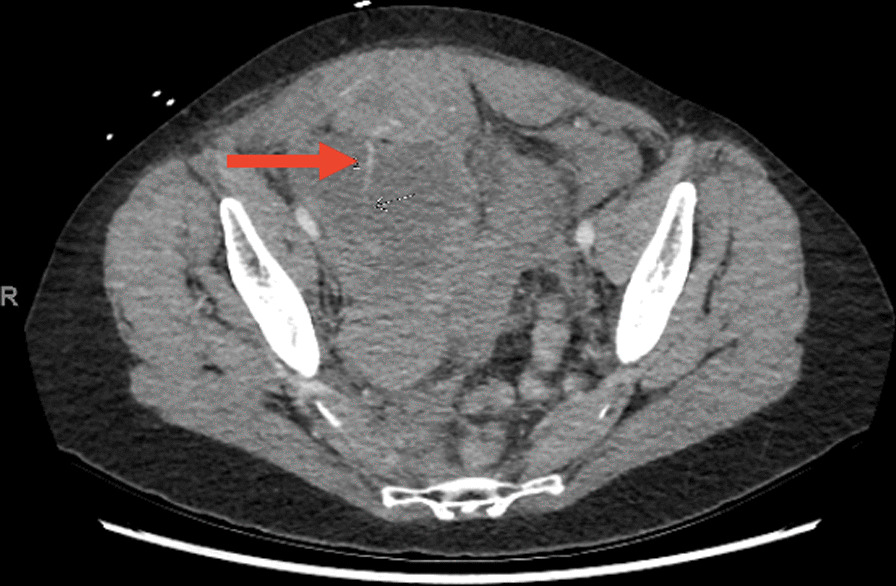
Axial slice of arterial phase contrast computed tomography abdominal scan showing a jet of active arterial bleeding (red arrow) from the right inferior epigastric artery
